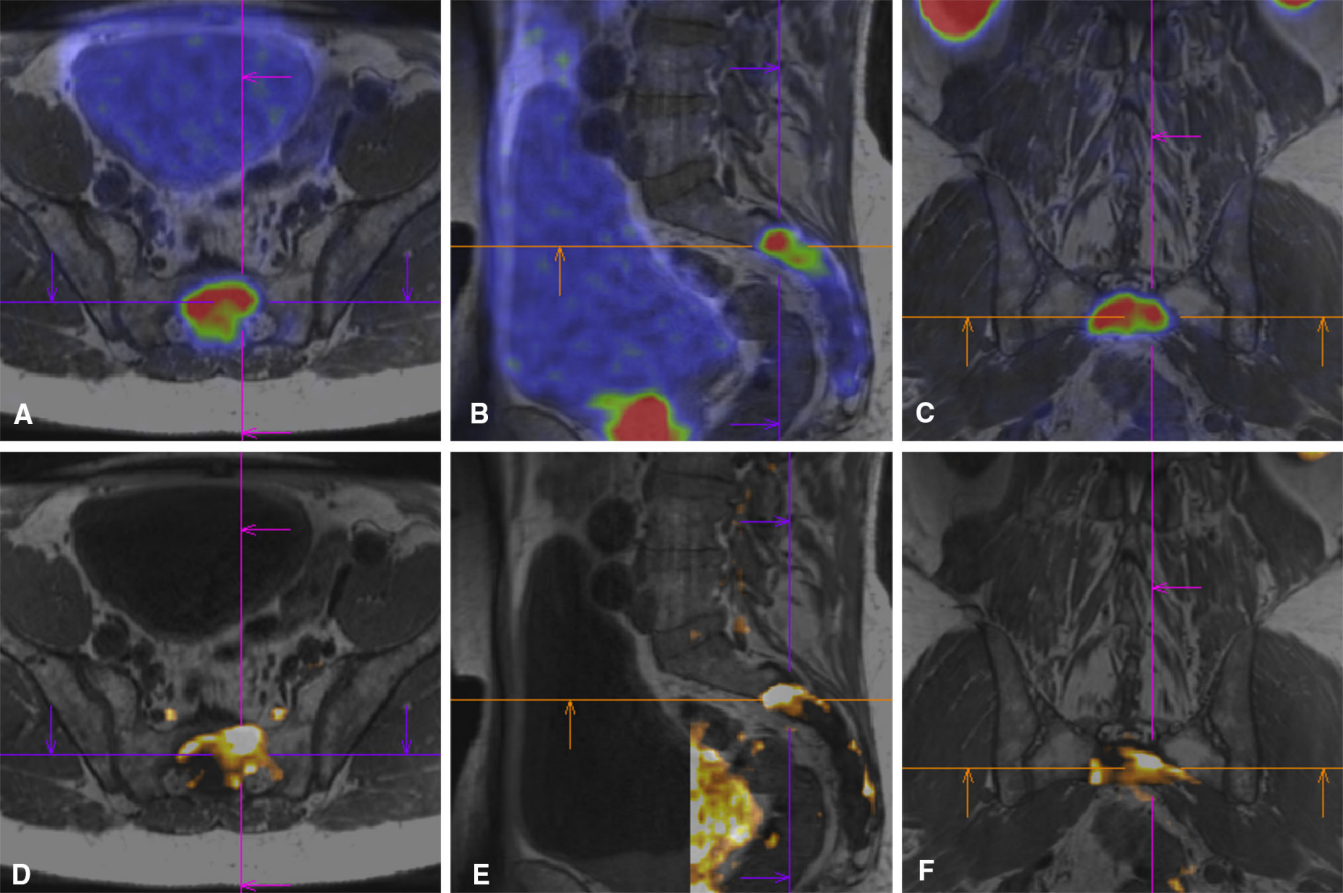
A–F Image fusion showed the difference in heterogeneity between PSMA uptake and diffusion restriction within some metastases. In this example of patient 8, the lesion in the sacrum appears larger on ^68^Ga-PSMA-PET than on DWI. A–C^68^Ga-PSMA-PET and D–F DWI images are both fused with the T1 MRI, and the lesion is visualized in (A + D) transversal, (B + E) sagittal and (C + F) coronal planes, respectively. The cross is centered at the same position in the T1 MRI for all images and can be used as a reference location. The target point was planned in the regional overlap between high PSMA uptake and high diffusion restriction
